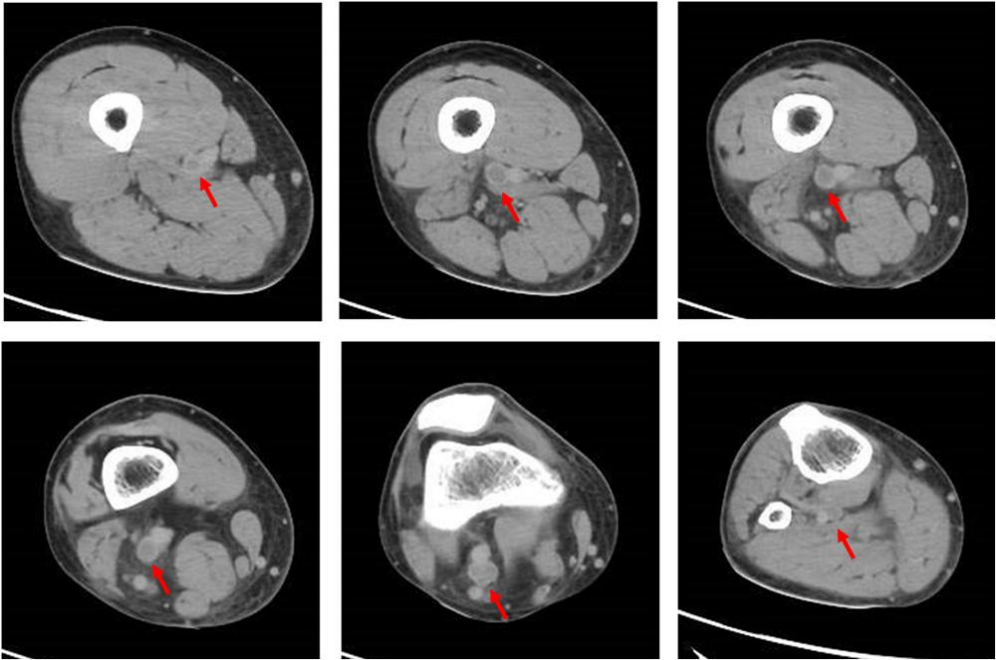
Venous phase of contrast-enhanced computed tomography. Thrombus was observed from the distal side of the right femoral vein to the deep leg vein (Red arrows).
